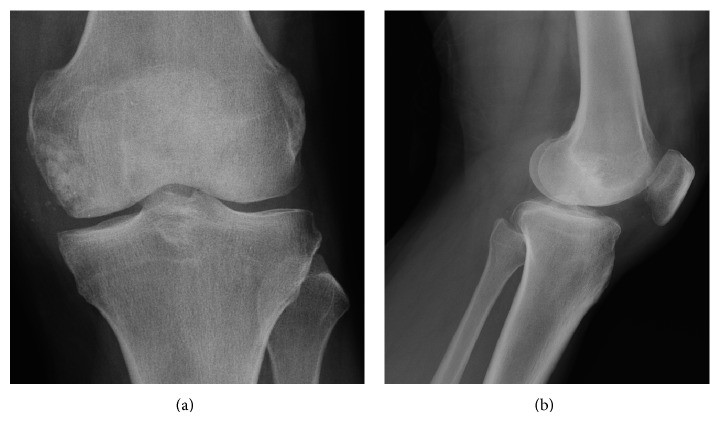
X-rays in AP (a) and profile (b) showing iliac bone graft placed in the site of the removal of the foreign body in the medial femoral condyle.
